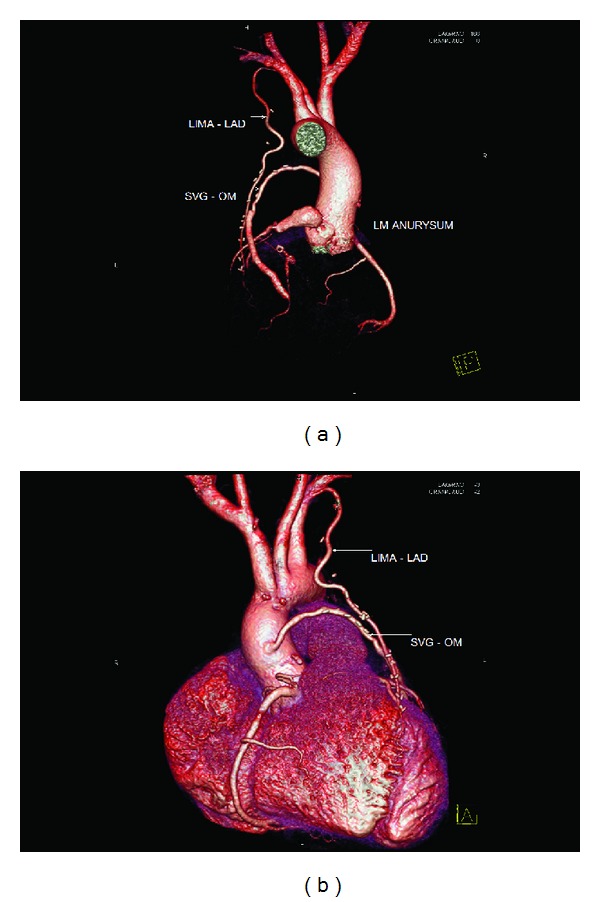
(a) Post-CABG CT Coronary angiogram showing proximally and distally ligated left main aneurysm with LIMA to LAD grafts and SVG to OM grafts. (b) Post-CABG CT Coronary angiogram showing proximally and distally ligated left main aneurysm with LIMA to LAD grafts and SVG to OM grafts.
